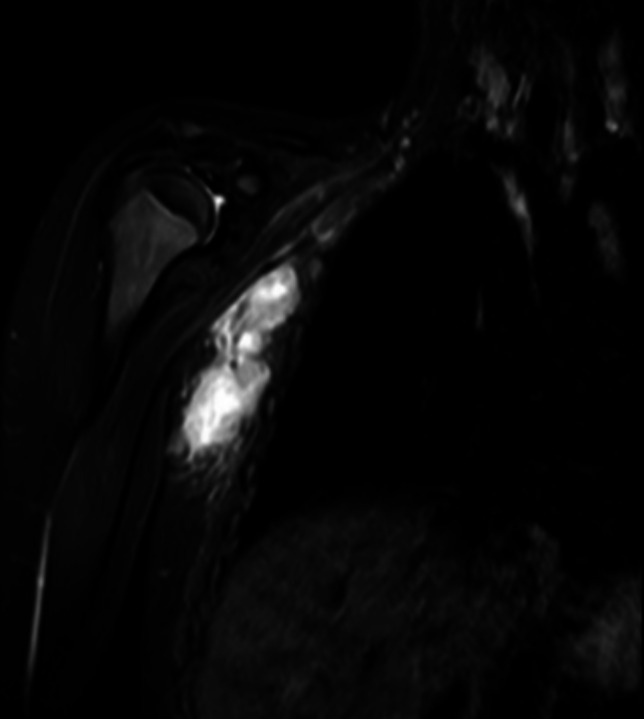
MRI imaging confirms the presence of multiple lymphadenopathy without malignant signs
